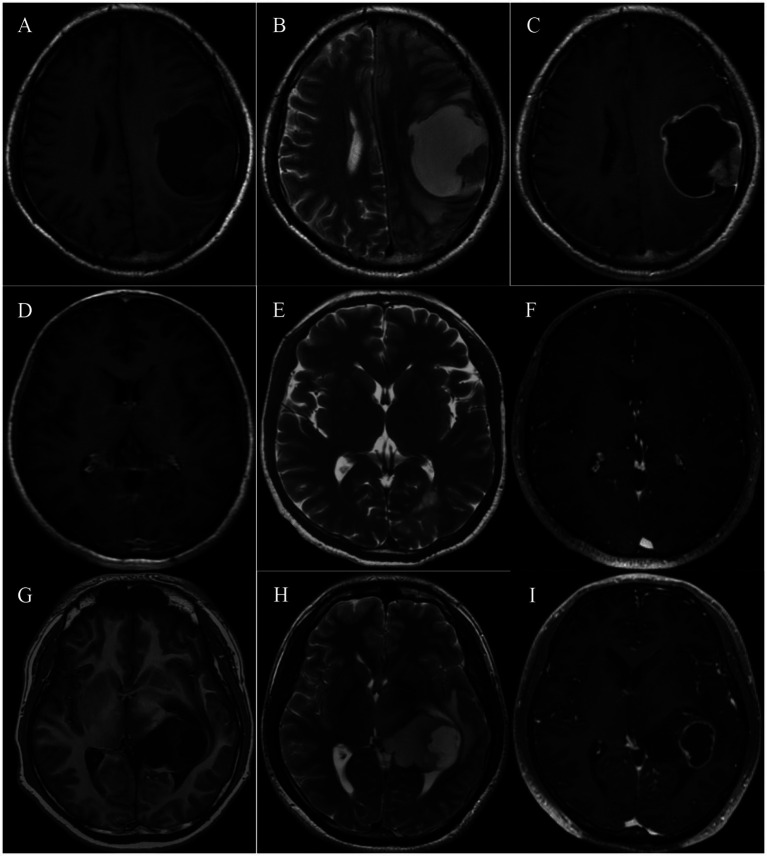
Typical MRI imaging of pediatric-type diffuse gliomas in adults. (A–C) MRI of a 32-year-old patient with primary diffuse pediatric-type high-grade glioma, H3-wildtype and IDH-wildtype located in the left frontal and parietal lobe. The lesion shows a mixed signal on T1 and T2-weighted imaging and consists of an intermediately enhancing nodule and a ring-like enhancing cyst cavity. (D–F) MRI of a 47-year-old patient with diffuse astrocytoma, *MYB*- or *MYBL1*-altered, located in the left occipital lobe. The restricted lesion is hypointense on T1-weighted imaging, hyperintense on T2-weighted imaging, and not enhanced. (G–I) MRI of a 25-year-old patient with diffuse midline glioma, H3 K27-altered, located in the left thalamus and lateral ventricle. The cystic-solid lesion shows a mixed signal on T1 and T2-weighted imaging and ring-like enhancement.
